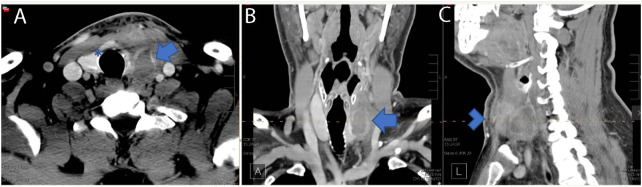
Four weeks post-RFA contrasted neck CT demonstrating a 3.3 cm × 2.1 cm × 3.5 cm (AP × TR × CC) low-density perithyroidal fluid collection with peripheral enhancement (arrow) deep to the sternocleidomastoid muscle with disruption of the anterior capsule (Asterix) associated with edema and reticulation of the overlying subcutaneous tissue and skin (arrowhead) consistent with nodule rupture. Features of the nodule rupture are shown in (A) transverse, (B) coronal, and (C) sagittal planes.
